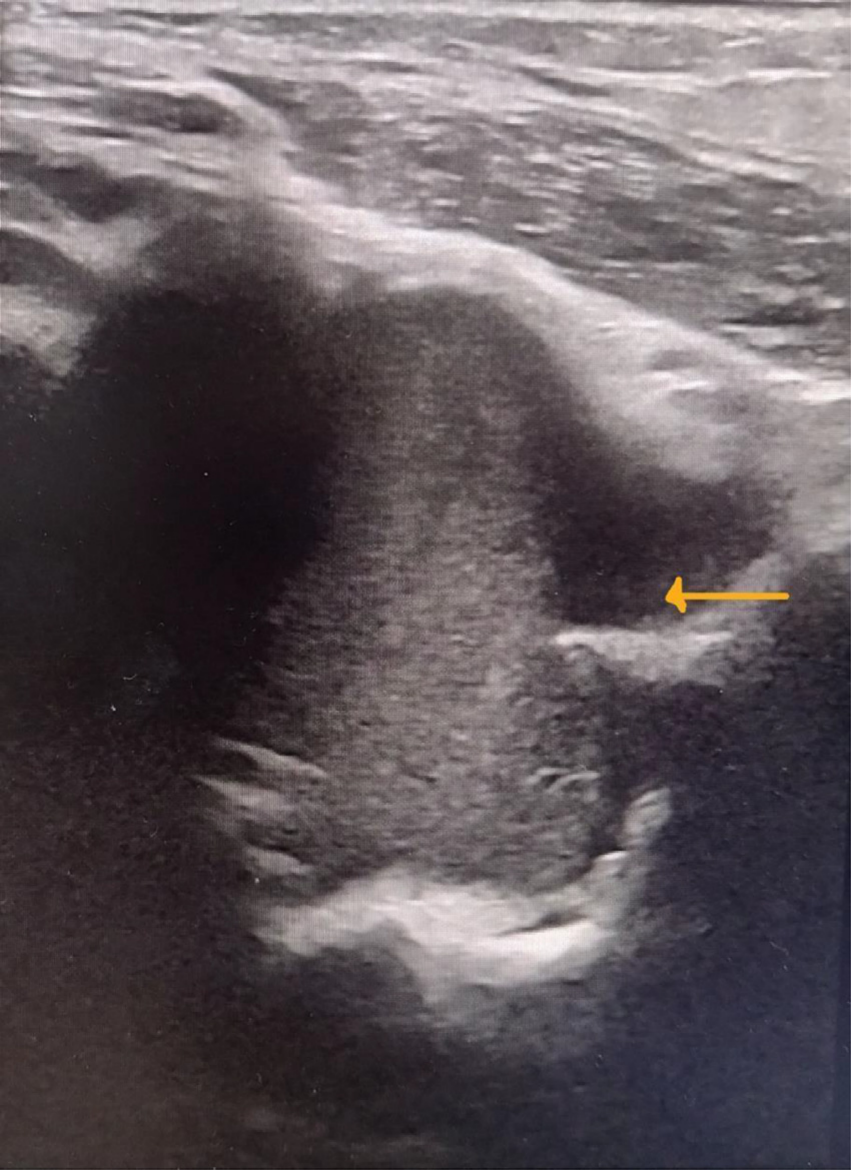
Ultrasound of left elbow joint. Yellow arrow points to joint effusion.
